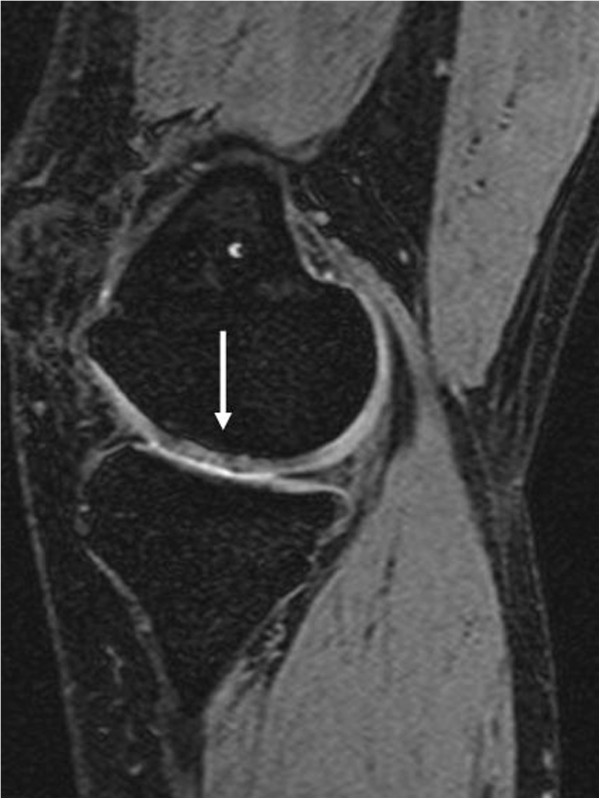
Follow-up MRI taken 36 months after surgery showing the cell-free scaffold (white arrow).
